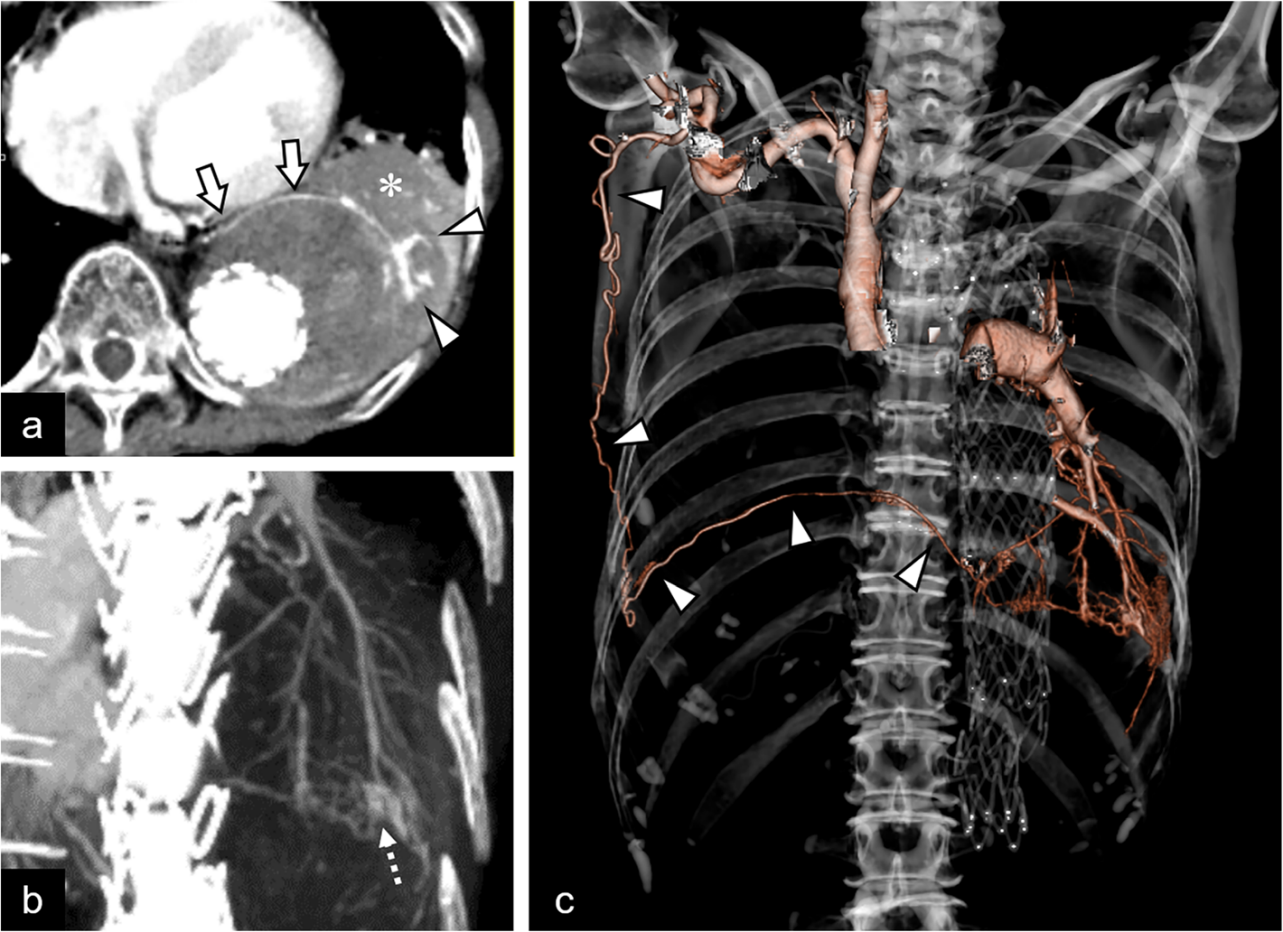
Computed tomography (CT) findings. A Contrast-enhanced CT showing the large atelectasis (asterisk) adjacent to a thoracic aortic aneurysm treated with a stent graft. The arrows and arrowheads indicate the hypertrophied left pulmonary ligament artery (PLA) and abnormal proliferative vessels in the atelectasis, respectively. B Slab-maximum intensity projection image in the oblique coronal view. Abnormal proliferation of small vessels is observed at the peripheral side of the left PLA. The dashed arrow indicates a pseudoaneurysm. C Volume-rendering image. The continuity from the right subscapular artery to the left PLA via the tortuous complex collateral pathway is clearly visible (arrowheads)
